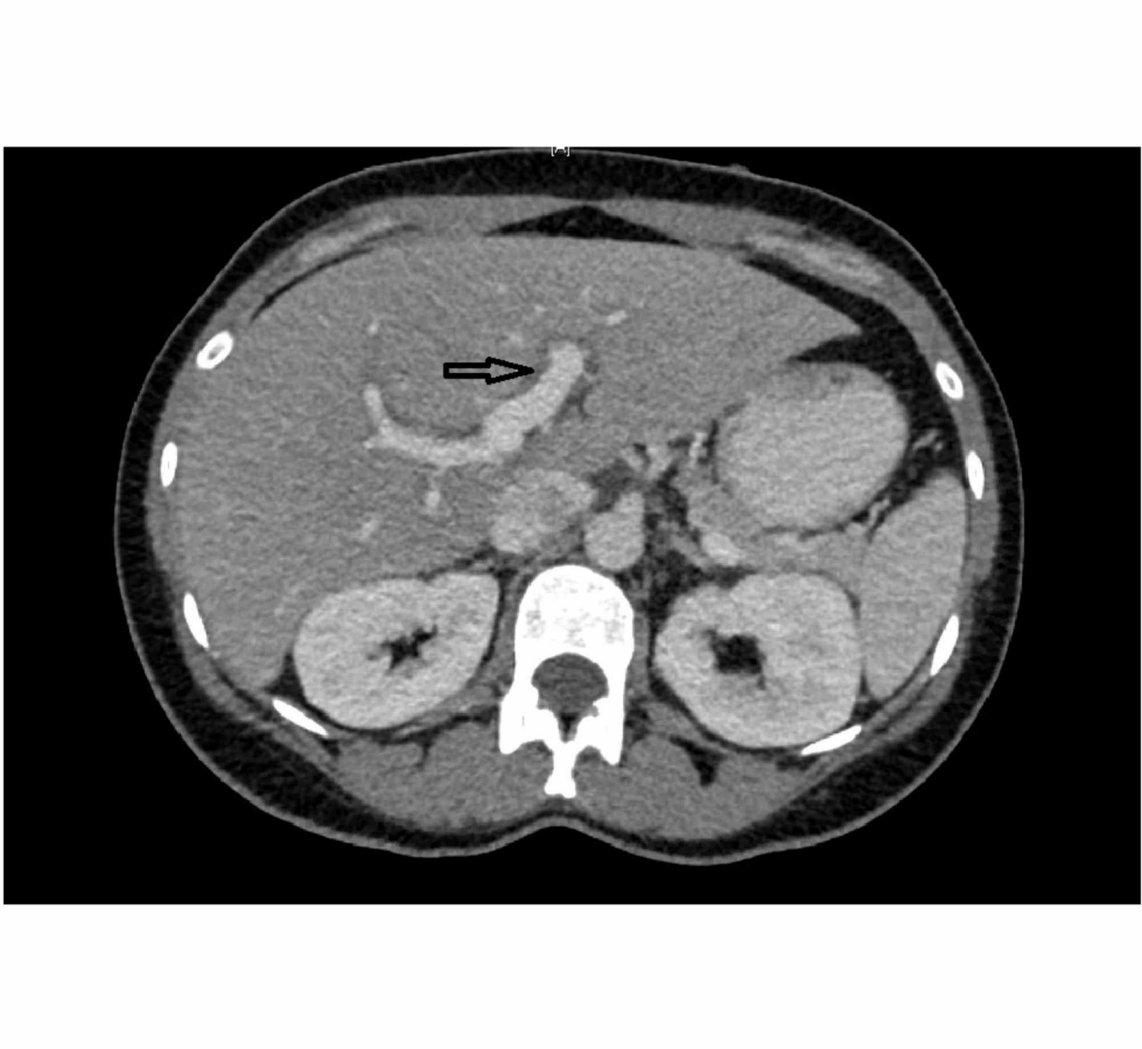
Cross-sectional view of CT scan of abdomen showing peri-portal edema (black arrow).
